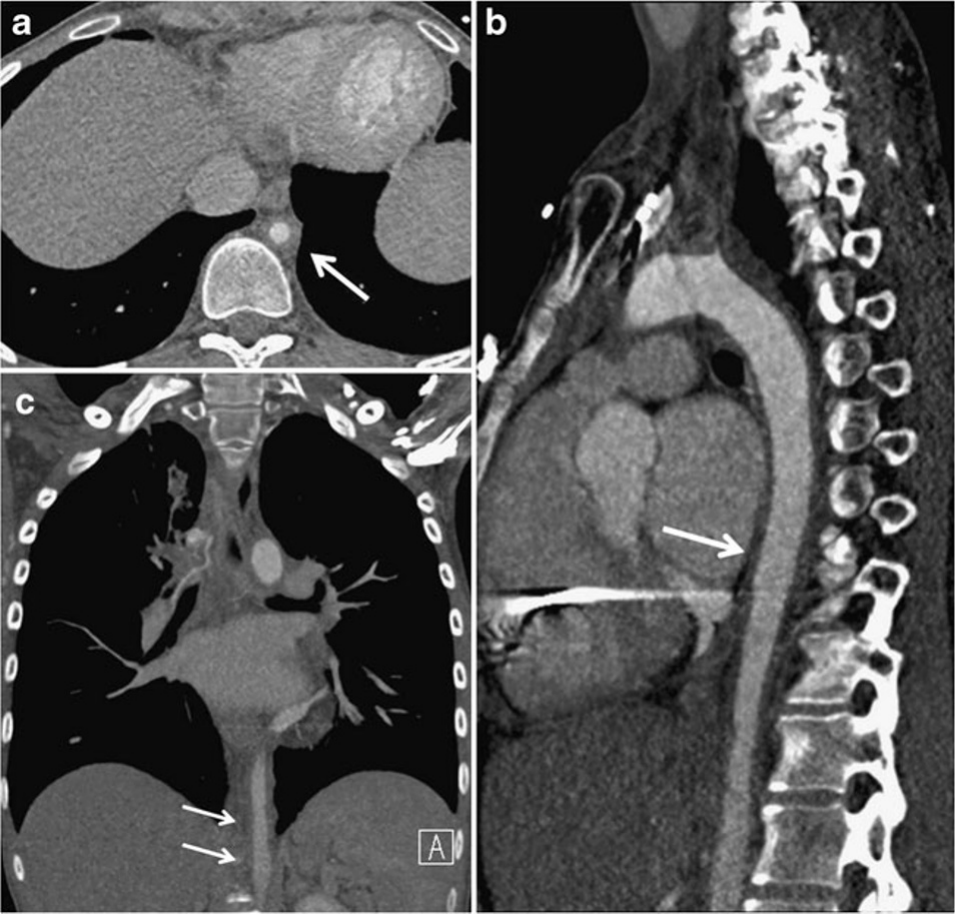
a–c A 25-year-old previously healthy man with chest and abdominal pain, weight loss and low grade fever. Axial (a), sagittal (b) and coronal (b) images of CTA of the torso demonstrate circumferential wall thickening of both the ascending and descending thoracic aorta as well as aortic wall thickening including in the abdominal aorta (*white arrows*). Diffuse narrowing of the aorta can be appreciated on all three views. Courtesy of Dr. Eduard Ghersin, Jackson Memorial Hospital, Miami, Florida
